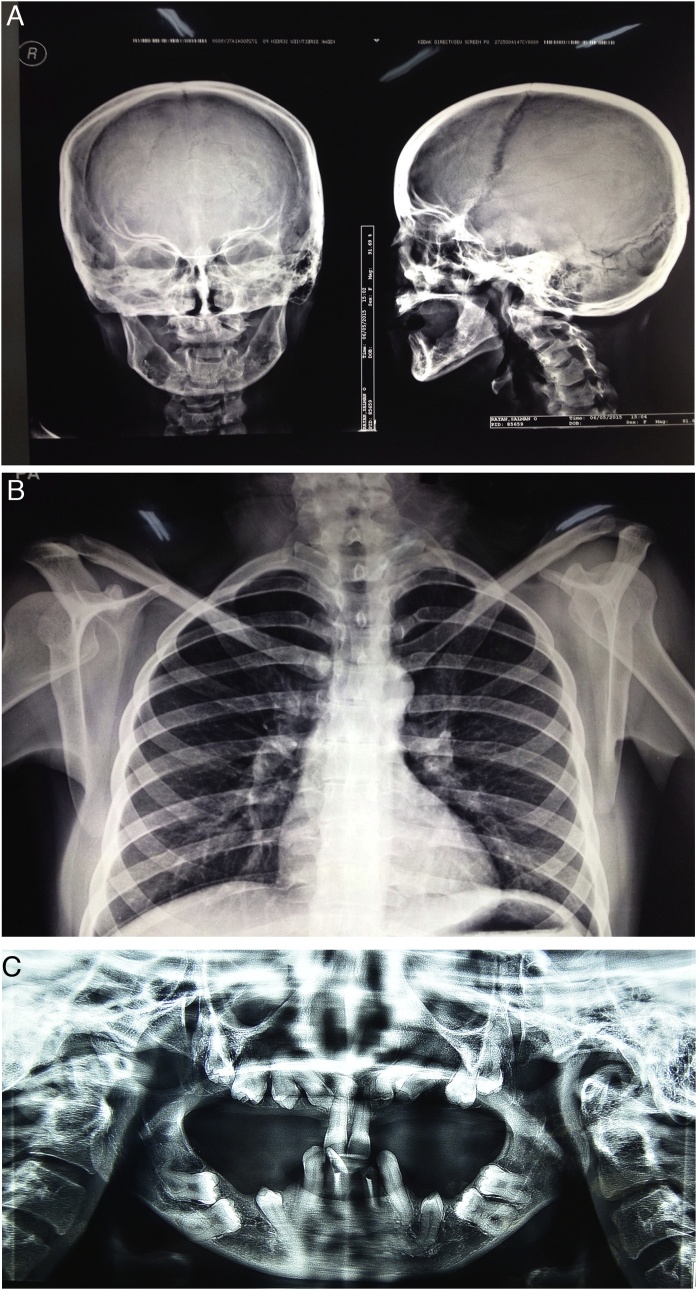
Preoperative radiographs of a 24-year-old woman with cleidocranial dysplasia. A. Anteroposterior and lateral skull views show small maxillary sinuses and thick cranial cortical bone with open sutures. B. A chest radiograph shows a bell-shaped thoracic cage and abnormally positioned clavicles. C. A panoramic radiograph shows multiple retained deciduous teeth, unerupted permanent teeth, and reduced maxillary bone height.
